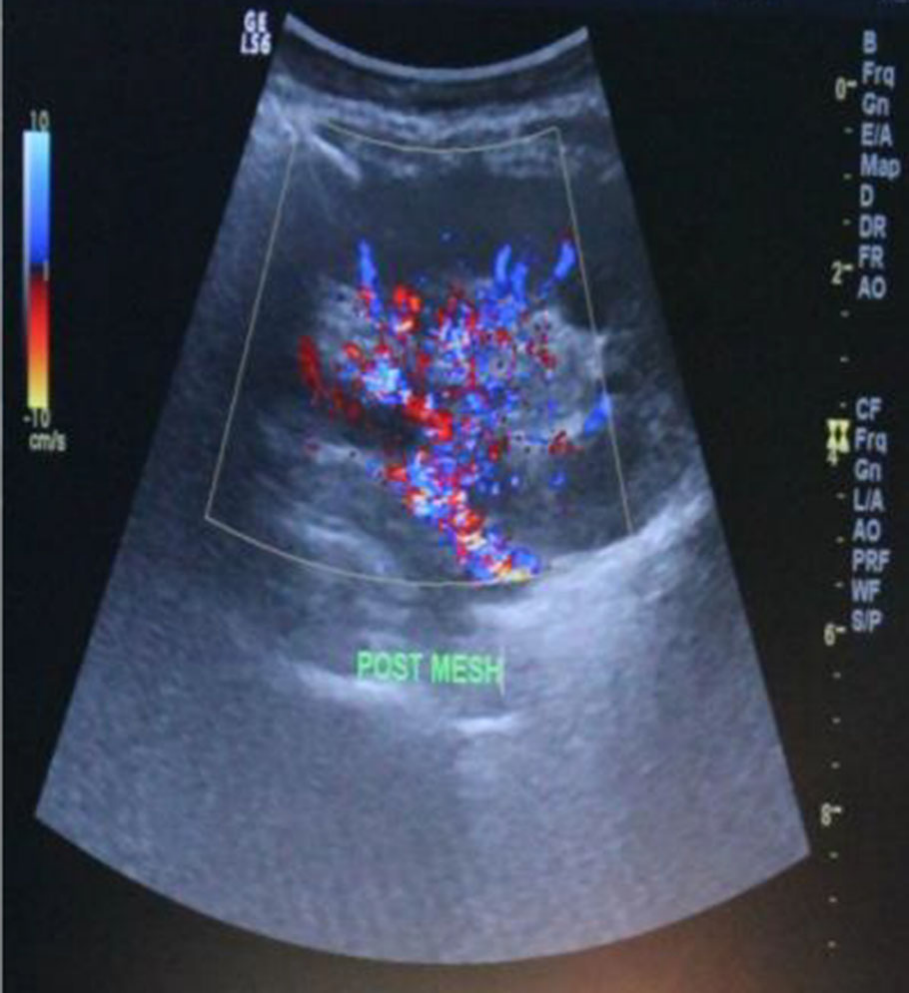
Ultrasound evaluation results of recipient after mesh insertion.
